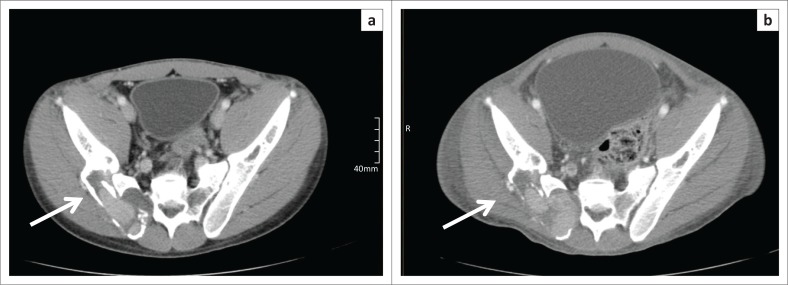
Restaging CT (a) Initial computed tomography (CT) (b) Right iliac skeletal lesion with a soft tissue component (arrow) where the soft tissue lesion proved difficult to measure reproducibly, resulting in significant inter-observer variability.
